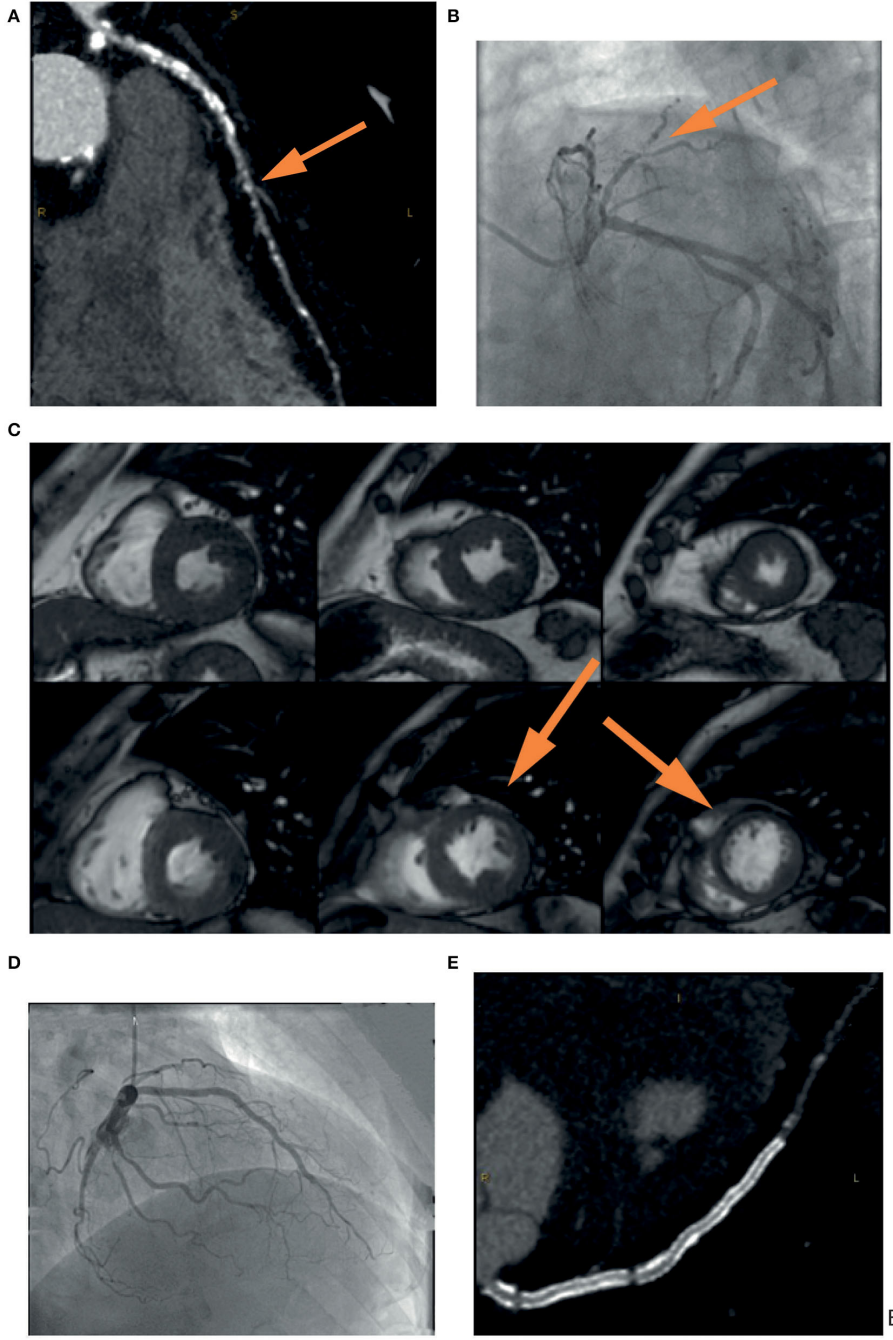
Example of the usefulness of multi-modality imaging in placing a correct indication for CTO percutaneous treatment. A 72-year-old patient performed a coronary computed tomography angiography (CCTA) (A) that showed severe three-vessel disease with occlusion (*yellow arrow*) of the left anterior descending (LAD) coronary artery. Coronary angiography (B) confirmed the presence of a calcified lesion occluding the LAD (*yellow arrow*) that was filled by means of collateral circulation. In consideration of the lack of symptoms and the unfavorable anatomy, it was decided not to proceed with a procedure without first assessing ischemia in the territory of the vessel. Cardiac stress magnetic resonance (C) showed inducible ischemia and myocardial viability in the mid-apical segment of the anterior wall and the interventricular septum (*yellow arrow*). Coronary angioplasty was then performed with implantation of a drug-eluting stent in the left main and LAD with an excellent result (D). Vessel patency without restenosis was confirmed by coronary CCTA at follow-up (E).
